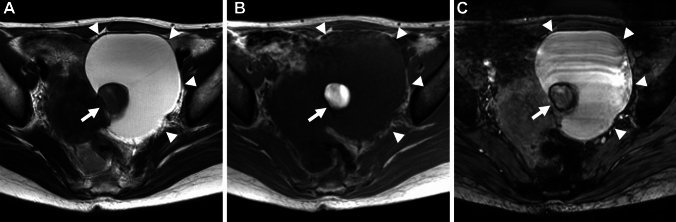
Peritoneal inclusion cyst associated with endometriosis. A 32-year-old with suspicion of a large ovarian cyst on ultrasonography examination. A T2-weighted image and, B T1-weighted image show a left small endometrioma (arrow) surrounded by a pseudocystic fluid collection (arrowheads) defined by the pelvic wall and pelvic organs. C SWAN revealed curved linear signal voids along the wall of the endometrioma
